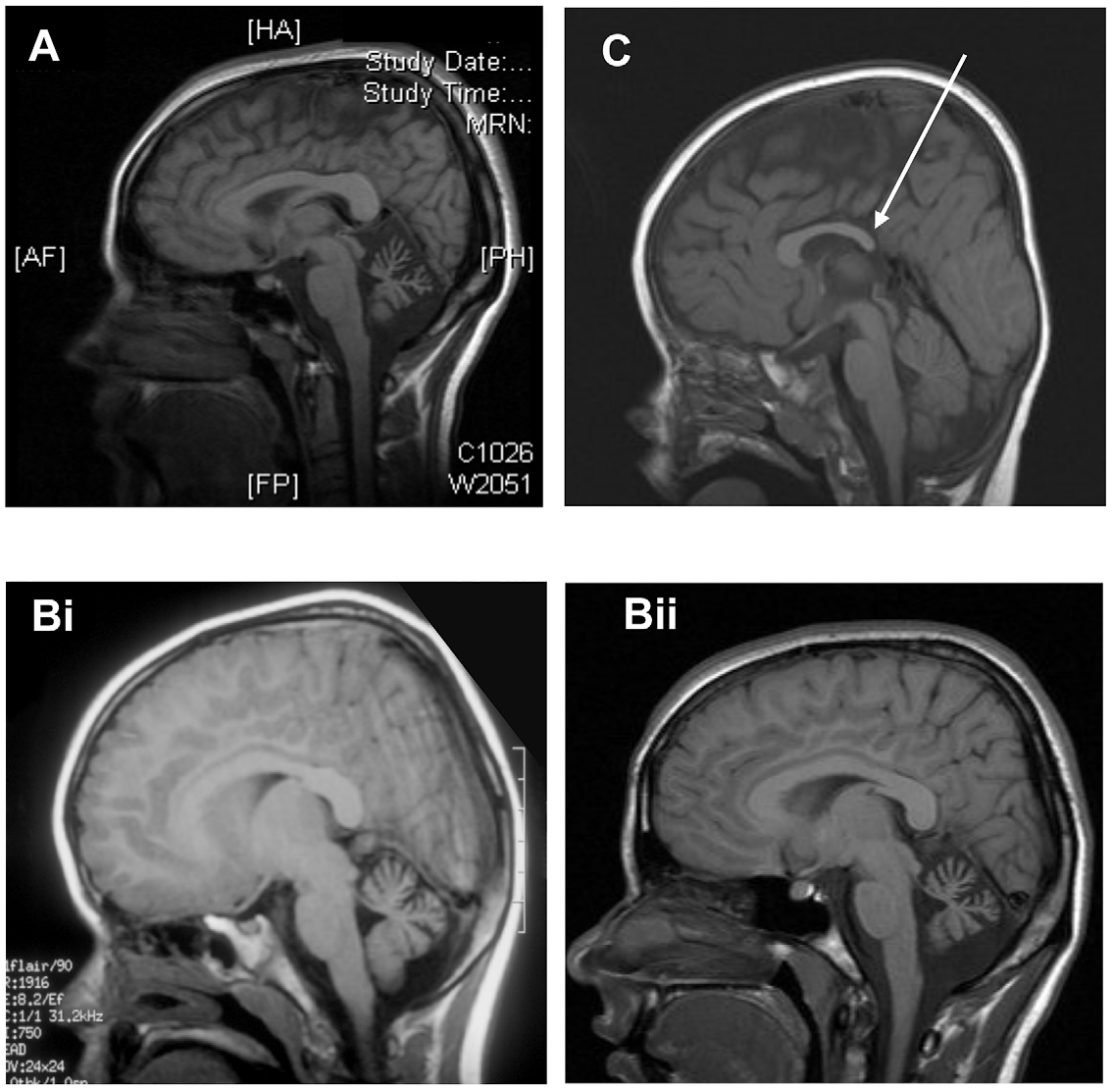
Neuroimaging of patients. A. Sagittal T1w MRI in subject V1 age 21 demonstrating clear cerebellar atrophy. B. Sagittal T1w MRI in subject V2 at age 6. Sagittal T1w MRI in subject V2 age 16 shows clear atrophy of the cerebellum. C. Sagittal T1w MRI in subject V3 showing hypoplasia of posterior corpus callosum (white arrow).
